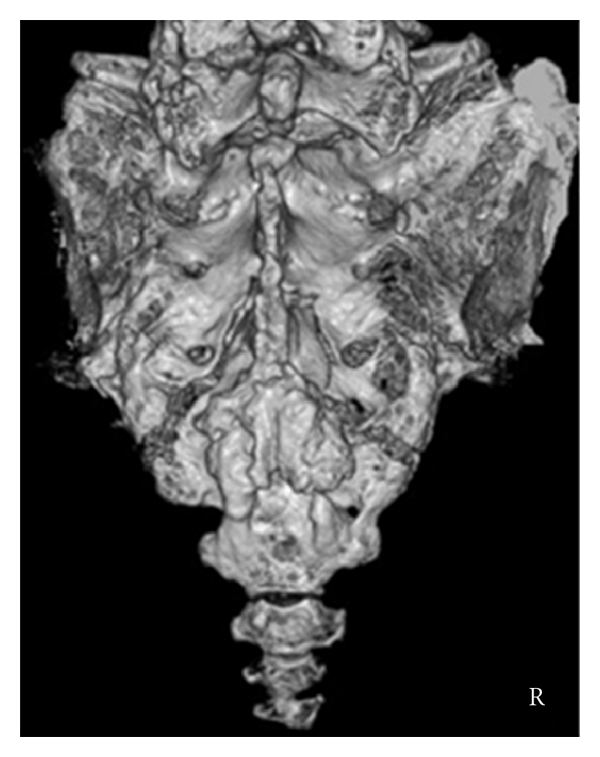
Preoperative three-dimensional CT: fracture lines began at the center of lamina and extended bilaterally.
